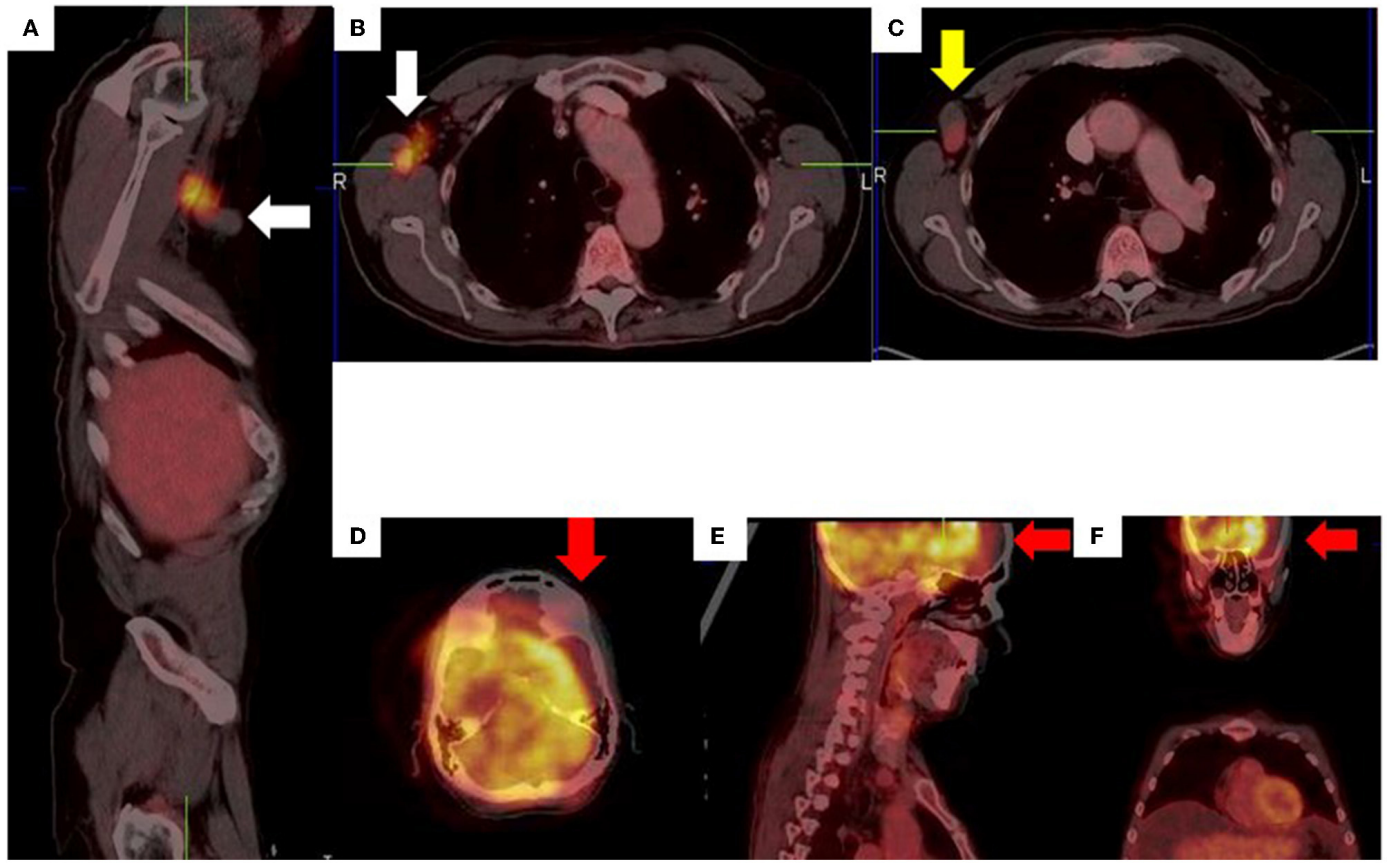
Right axillary lymph node misregistration artifact due to limb motion (A–C). Intense uptake in the right axilla does not conform to a specific anatomical structure (white arrows) while the axillary lymph node demonstrates low grade uptake (yellow arrow). Misregistration between PET and CT images (D–F) due to voluntary head motion.
